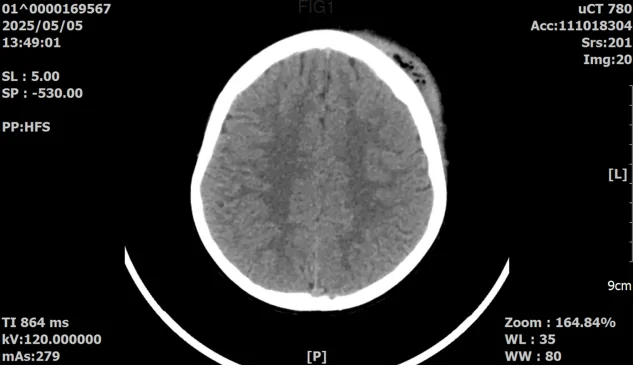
Cranial computed tomography scan showing subcutaneous air and a small hematoma in the left frontal surgical area (yellow arrows). No skull destruction, intracranial extension, or abscess formation is observed.
